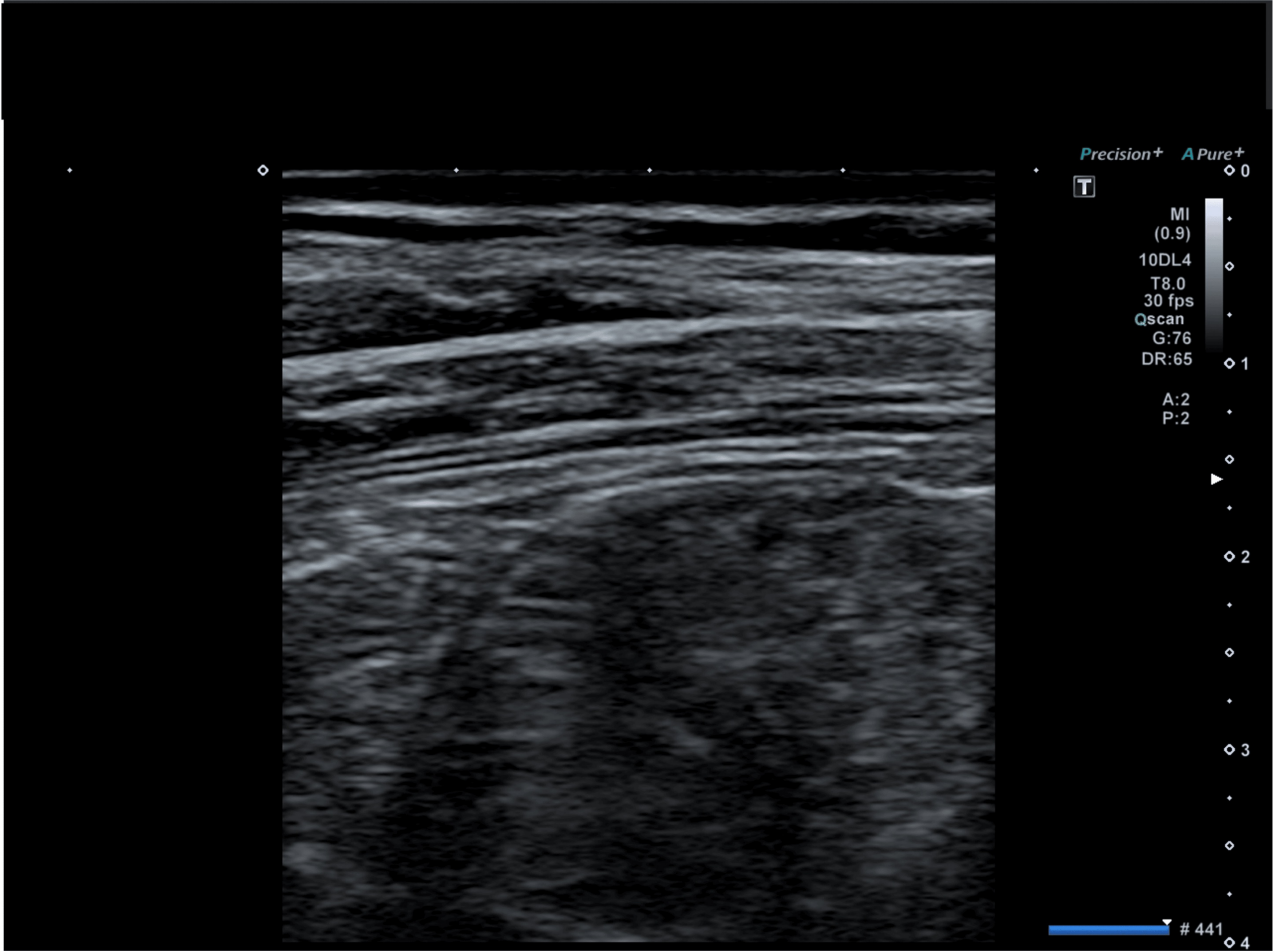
Ultrasonography image. A mass or tumor was not detected at the affected site.
